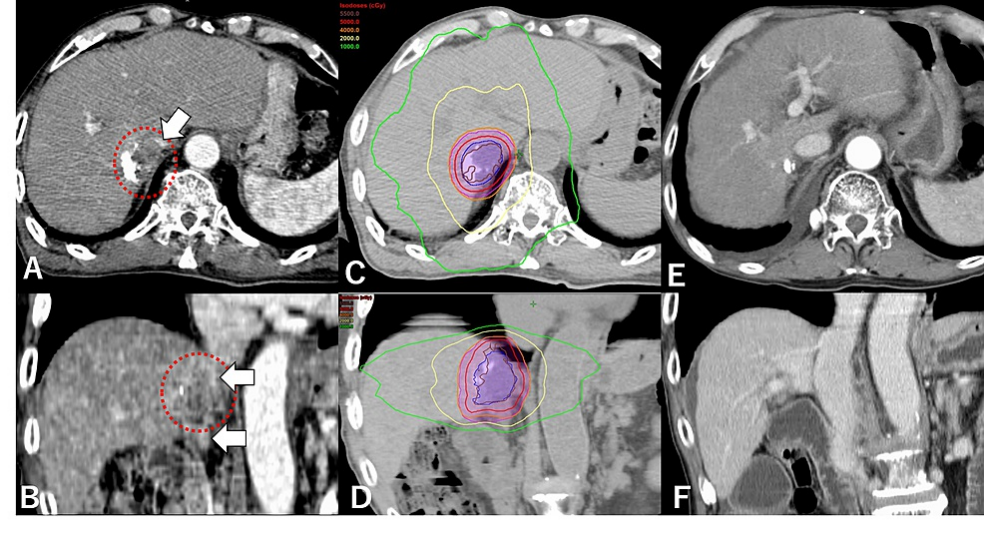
Case 2 – salvage SBRT for lesion unresponsive to TACE A, B: treatment planning CT of the liver demonstrating hepatocellular carcinoma (dotted line) invading the inferior vena cava (IVC) (arrows). C, D: axial and coronal images of the dose distribution of radiotherapy. SBRT was performed with 40 Gy in five fractions prescribed to enclose the planning target volume with a 70% isodose line of the maximal dose equated to the prescribed dose. E, F: the latest CT with no recurrence 22 months after SBRT. The IVC was kept flowing. The patient died of pneumonia two months after this scan SBRT: stereotactic body radiotherapy; TACE: transcatheter arterial chemoembolization; CT: computed tomography
